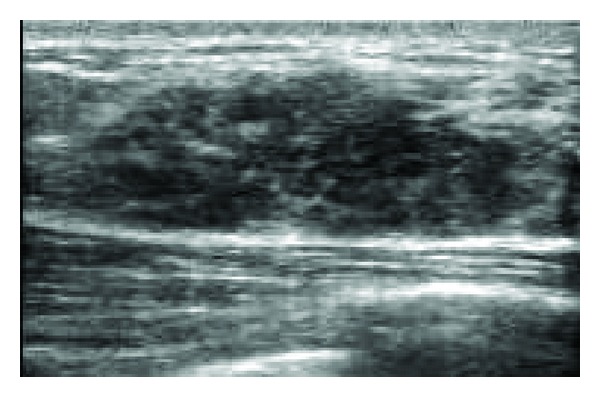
Ultrasonography showed a heterogeneous hypoechoic mass with an ill-defined margin, measuring 3.4 × 1.1 × 3.0 cm.
